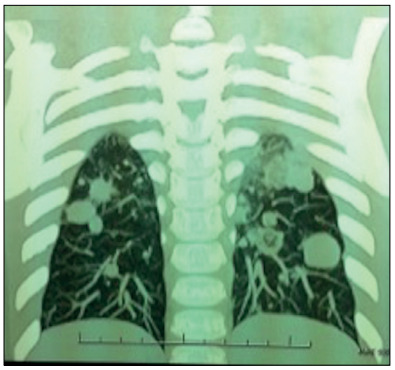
First pulmonary computed tomography scan showing numerous bilateral solid nodules, in the patient at the age of three years.
